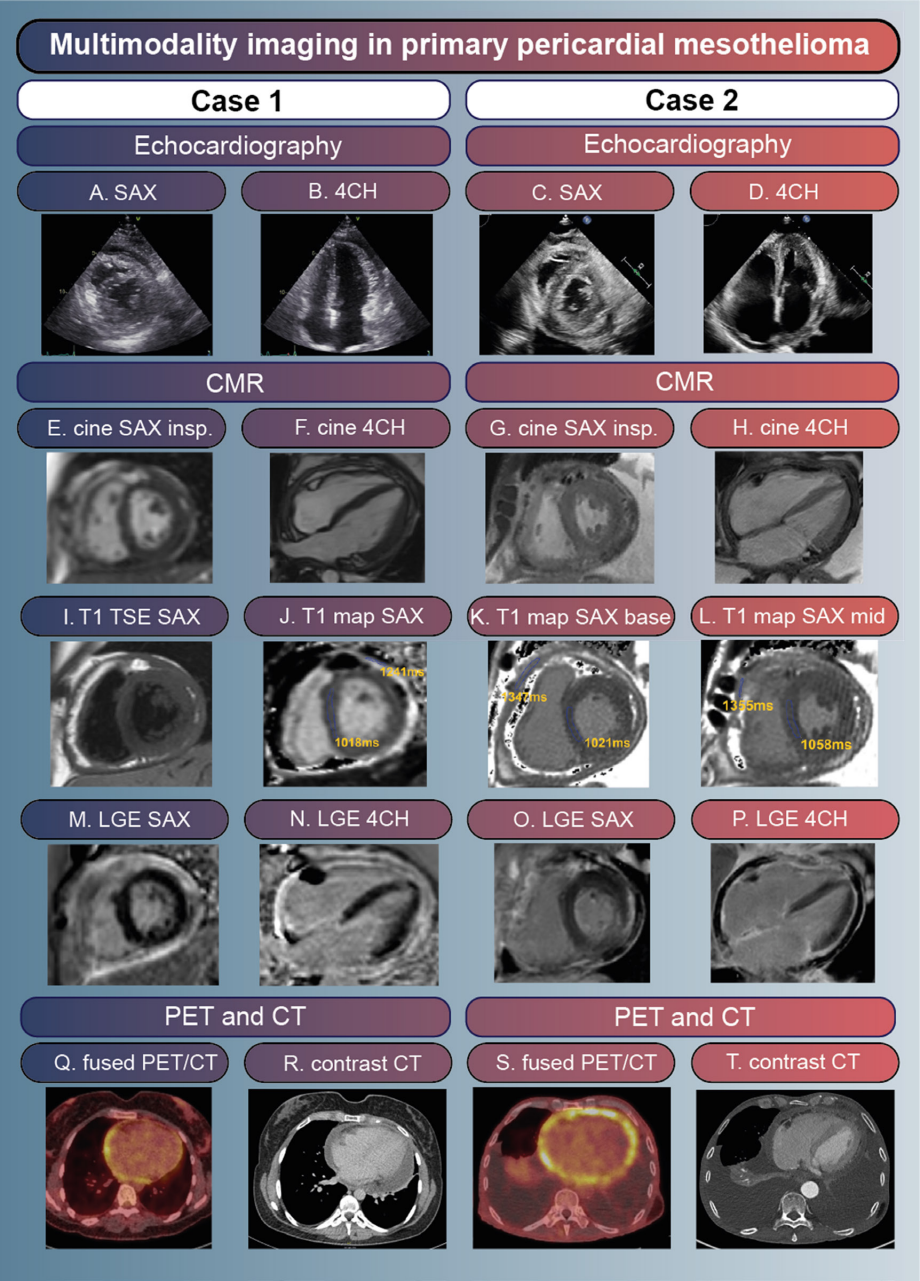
Multimodality imaging by echocardiography, cardiac magnetic resonance (CMR), computed tomography (CT), and positron emission tomography (PET)/CT. A to D, Leftward interventricular septum (IVS) shift on inspiration. C and D, Right-sided dilation and pleural effusion. E and G, Leftward IVS shift on inspiration. F and H, Mild pericardial effusion, adhesions and thickened pericardium. I, Thickened pericardium. J to L, Prolonged native T1 values in pericardium, normal native T1 values in myocardium. M to P, High signal intensity of pericardium. Q and S, High fluorodeoxyglucose uptake in the pericardium with a maximum standardized uptake value (SUV_max_) of 6.28 and 8.74, respectively, for case 1 and 2. R and T, Contrast-enhanced chest CT showed no pericardial calcifications. 4CH indicates four chamber; LGE, late gadolinium enhancement; SAX, short axis; and TSE, T1-turbo spin echo.
